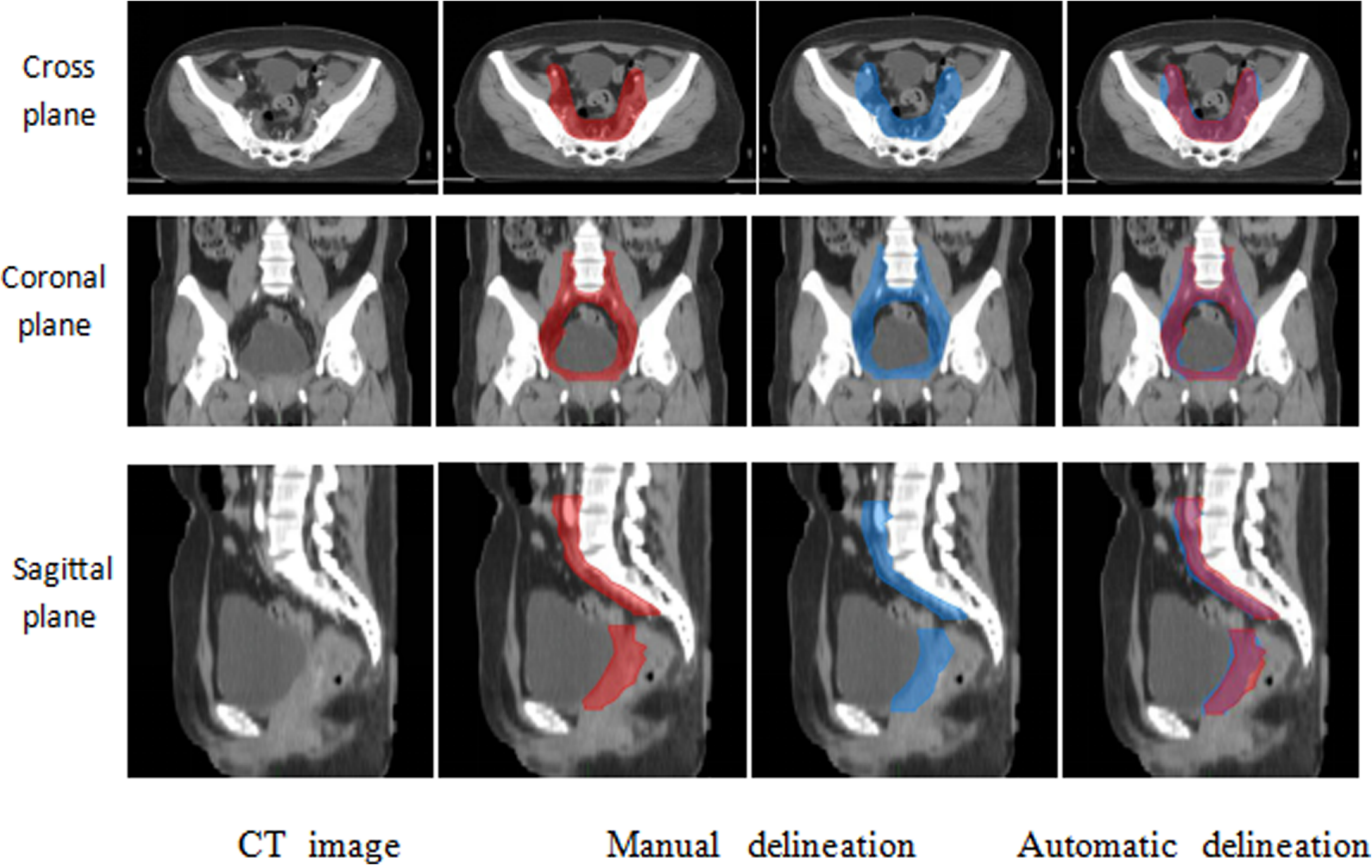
Patient CT image and sketching results
